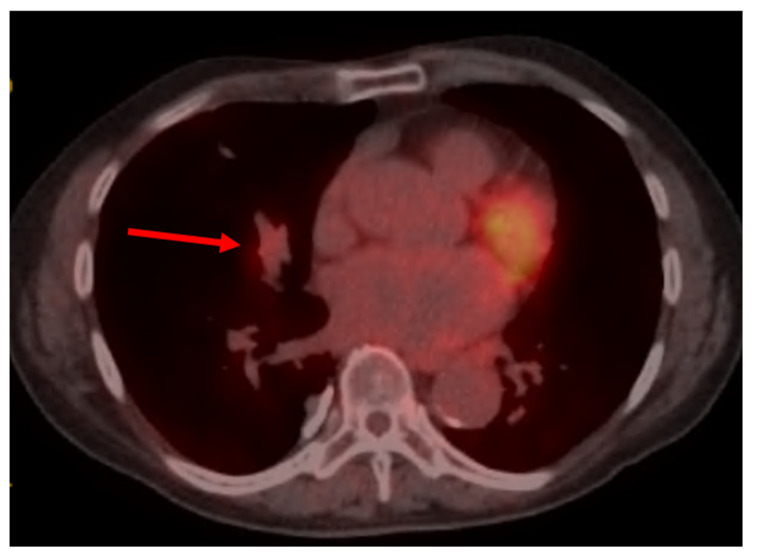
PET/CT scan with a red arrow pointing to the right middle lobe opacity and atelectasis extending from the perihilar region to the periphery of the lung, with mild uptake (SUVmax 2.7) at the perihilar region, suggestive of mucoid impaction versus low-grade endobronchial malignancy.
